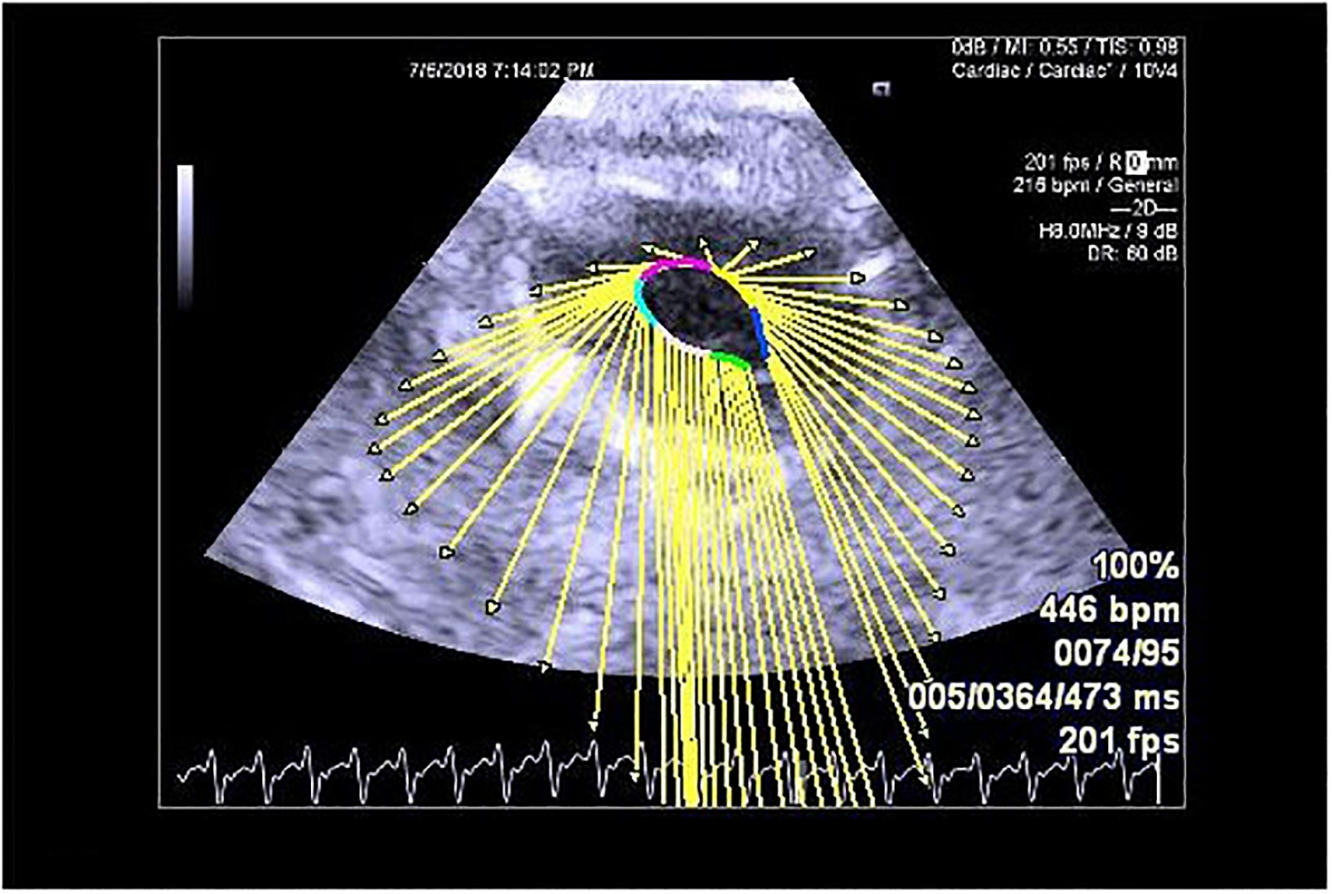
VVI technology to analyze the apical four-chamber view of the left ventricle.
